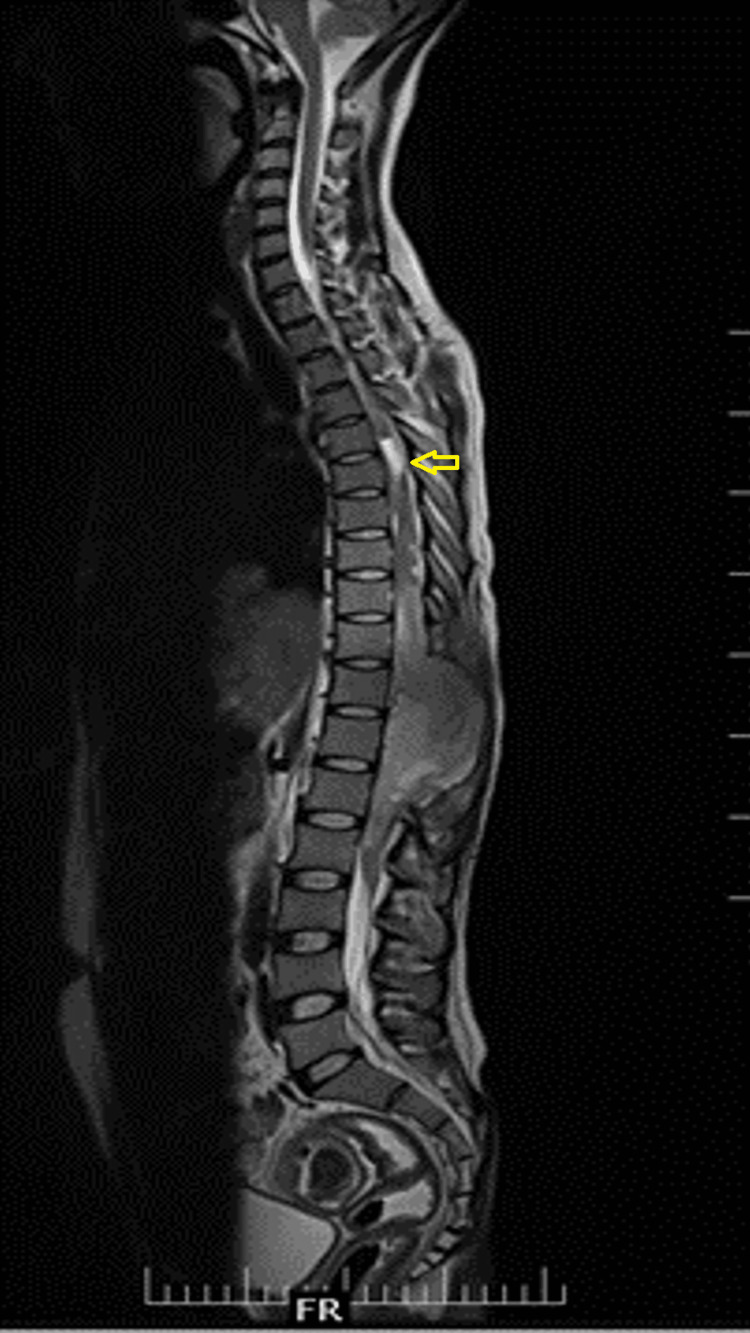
An MRI of the whole spine screening (T2-weighted mid-sagittal image) shows cord edema ascending to the T6 level (yellow arrow).
